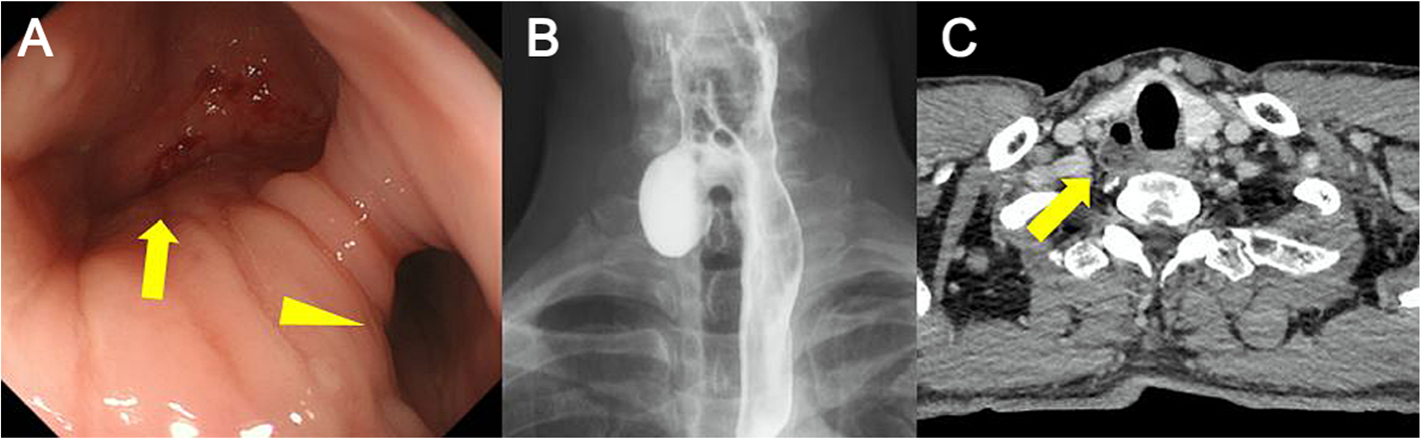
a Upper intestinal endoscopy shows a diverticulum (yellow arrow) and the true lumen of the esophagus (yellow arrowhead). b Esophagogram shows a right-sided outpouching sac from the esophagus. c Computed tomography with contrast shows diverticulum from the esophagus at the level of the cricothyroid cartilage
